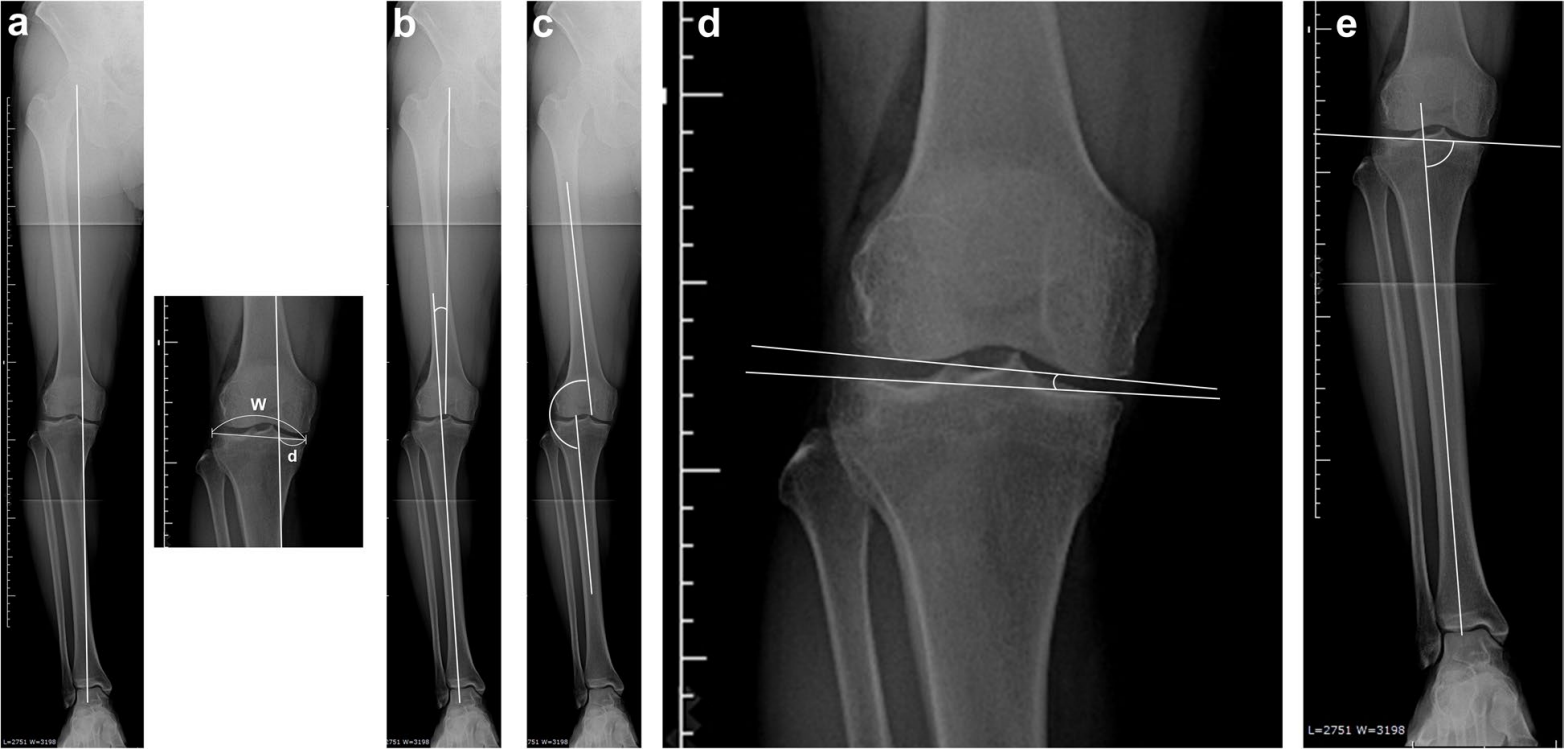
Radiographic evaluation (bilateral standing whole-leg radiographs), a The weight-bearing line ratio (WBL ratio). The horizontal distance from the medial edge of the tibial plateau (d), divided by the width of the tibial plateau (W), as d/W × 100%. The WBL ratio of the medial edge was regarded as 0% and the lateral edge as 100%, b Hip-knee-ankle angle (HKA), the angle formed by the mechanical axis of femur and tibia. Negative values are assigned to knees with varus alignment, c Femoro-tibial angle (FTA), d Joint line convergence angle, e Medial proximal tibial angle
